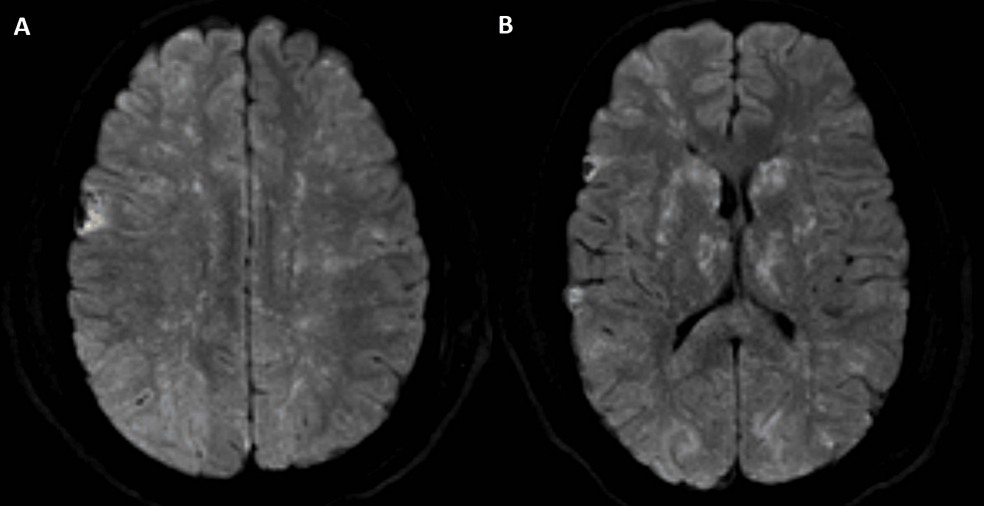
Axial slices of magnetic resonance imaging of the head with diffusion-weighted imaging demonstrating a diffuse starfield pattern of diffusion restriction, a hallmark finding of cerebral fat embolism syndrome.
